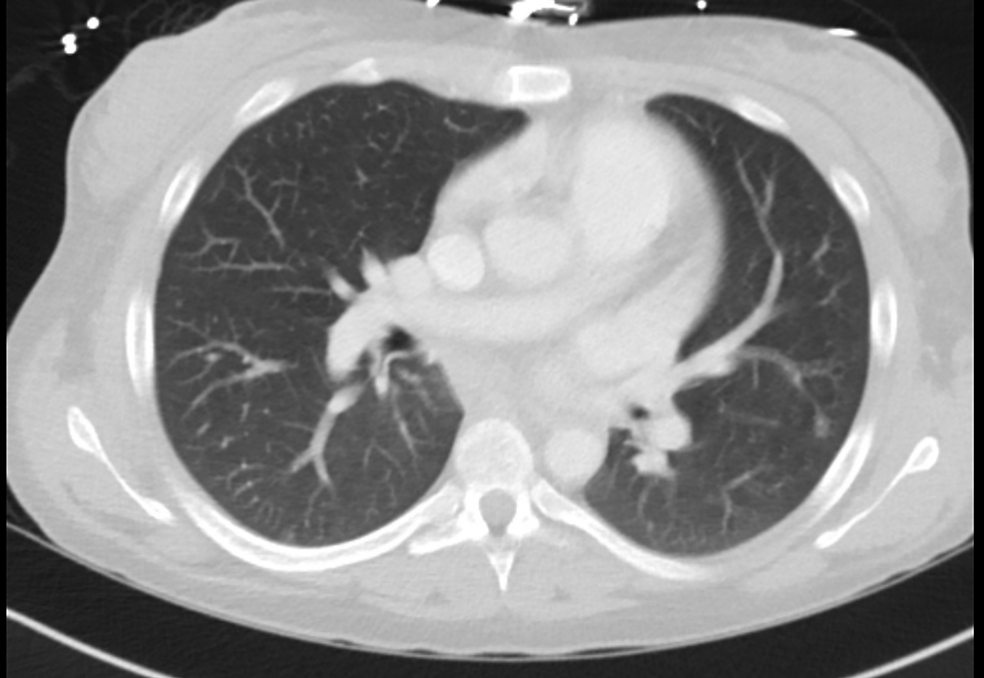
CT chest with contrast was unremarkable for pulmonary embolism
